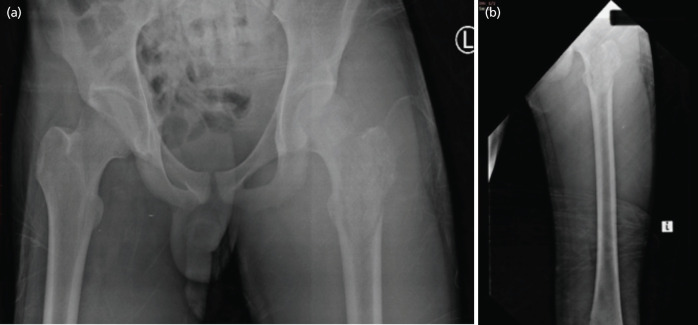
(a) AP view of pelvis and (b) lateral radiograph of left femur showing sclerosis of the greater trochanter of left femur.
